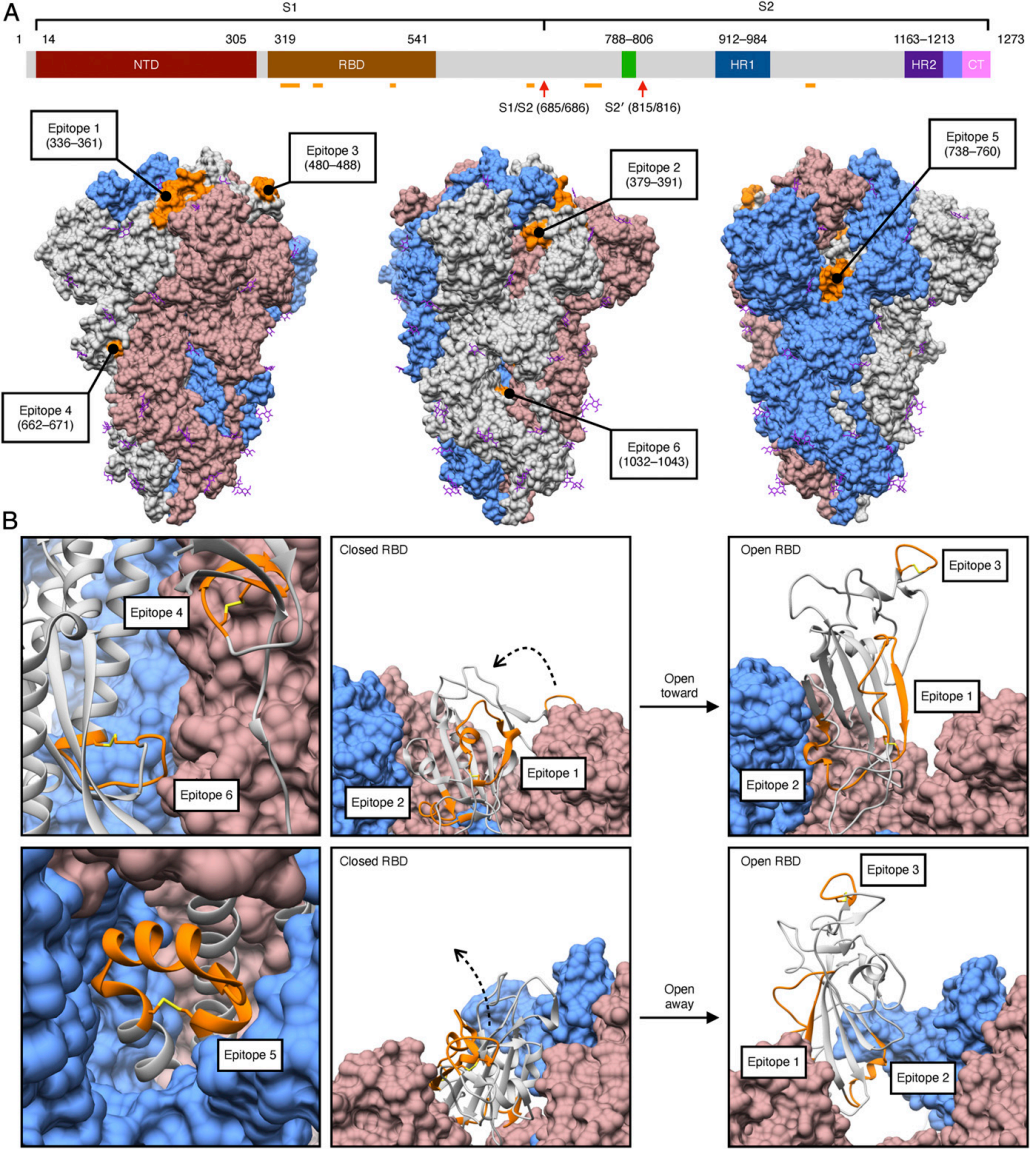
Identification of structural epitopes on the S protein trimer. (*A*) Six epitopes (orange) spanning the SARS-CoV-2 S protein were selected for display on the rpVIII protein. Four epitopes are located within the S1 subunit, epitope 1 (aa 336 to 361), epitope 2 (aa 379 to 391), epitope 3 (aa 480 to 488), epitope 4 (aa 662 to 671), and two within the S2 subunit, epitope 5 (aa 738 to 760), and epitope 6 (aa 1,032 to 1,043). These epitopes are solvent exposed in the surface representation of the predominantly closed-state conformation of the S protein trimer (gray, cornflower blue, and rosy brown) (PDB ID: 6ZP0) (63). Glycans (shown in purple) are present throughout the structure; of the six epitopes, only epitope 1 (aa 336 to 361) contains a site for glycosylation (at N343). Two cleavage sites located in the S2 subunit, S1/S2 (aa 685/686) and S2′ (aa 815/816), are represented by red arrows. (*B*) All of the epitopes (orange) maintain a cyclic conformation in the ribbon representation of a S protein protomer (gray); disulfide bridges (yellow) are present between the flanking cysteine residues of all epitopes except on epitope 2 (aa 379 to 391). The open-state conformation of the S protein trimer with one RBD erect displays a change in orientation of epitopes 1, 2, and 3, though all remain solvent exposed (PDB ID: 6ZGG) (64). NTD, N-terminal domain; RBD, receptor-binding domain; HR1 and HR2, heptapeptide repeat sequence-1 and -2; CT, C terminus.
